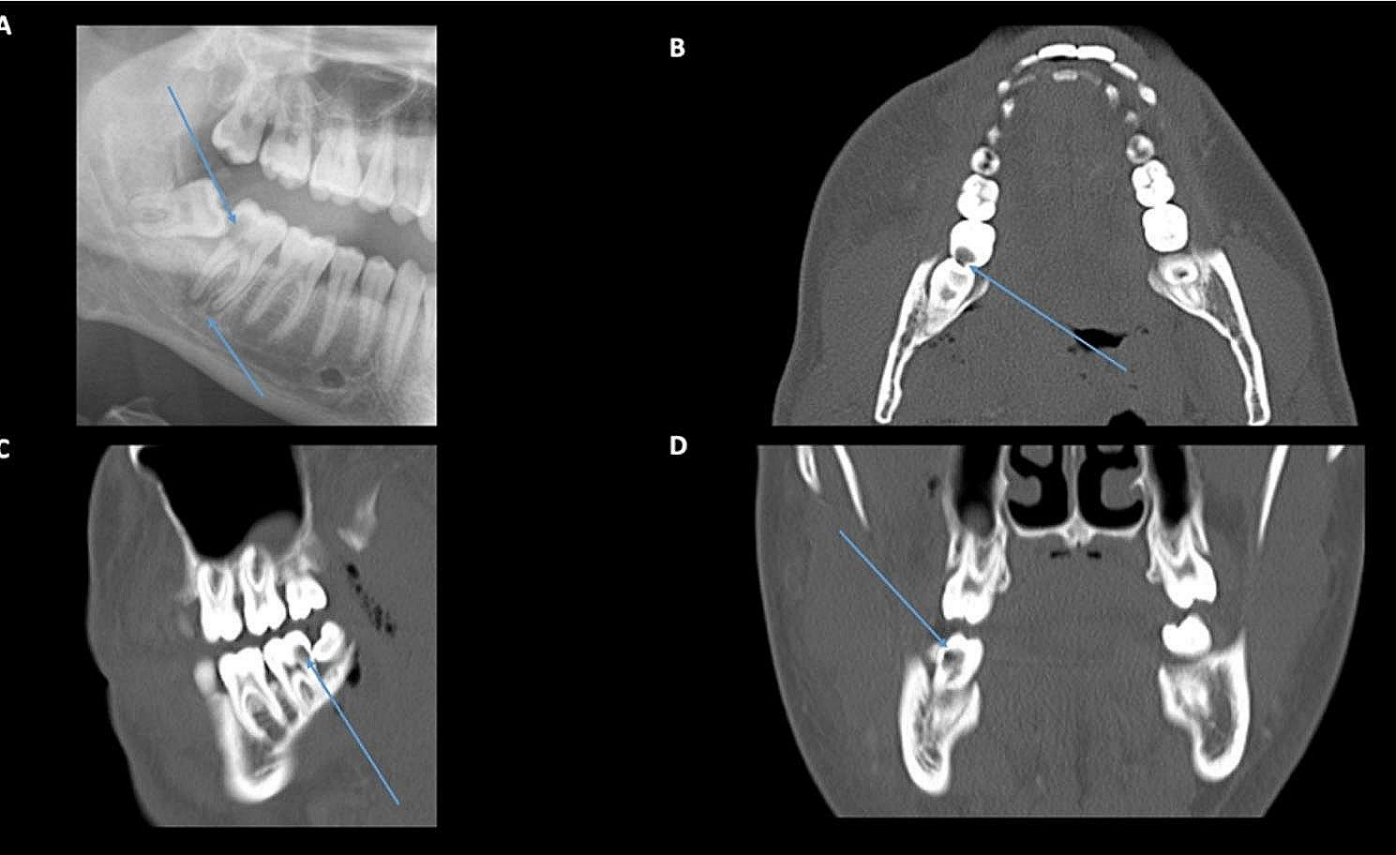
Case 1: OPG and CT facial bone scans. A: OPG image showing extensive carious damage on the crown of the lower right molar (47) and periapical radiolucency suggesting infectious spread through alveolar bone (arrows). B-D: There is extensive radiolucency on 47 distal
